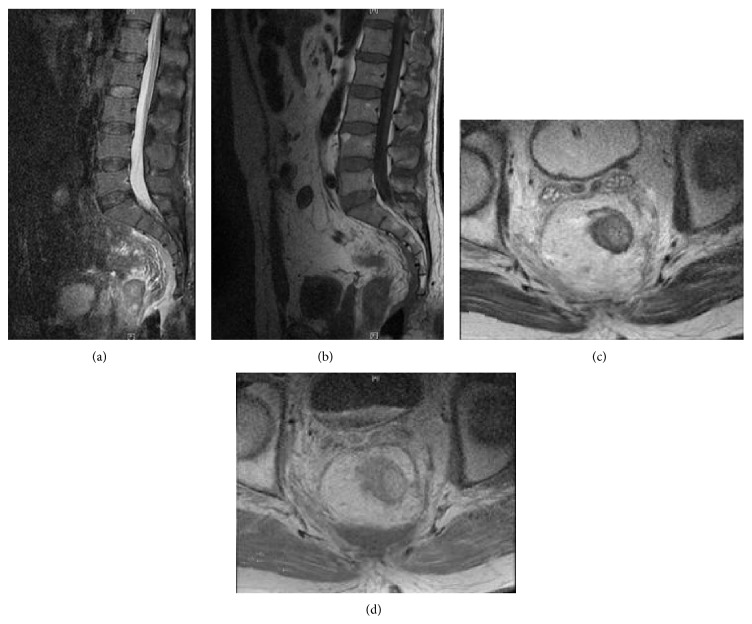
Sagittal and axial MRI following the removal of the mass.
